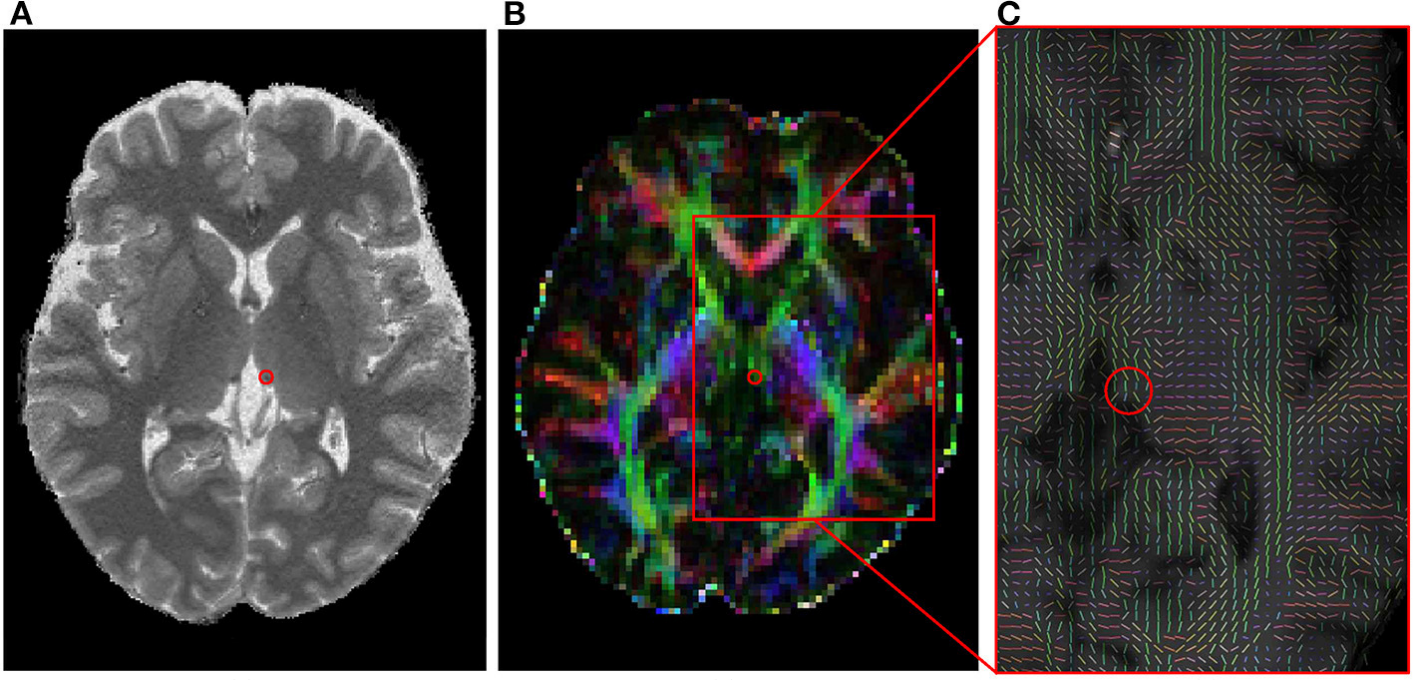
The habenula in a 3T MRI image and its diffusion tensor image. (A) A selected T1 image indicating a habenula area with a red circle. (B) A fractional anisotropy image corresponding to (A). (C) A zoomed fiber orientation image estimated with BEDPOSTX. The mean principle diffusion direction distribution in the red box of (B) was shown in a vector form. MRI, magnetic resonance imaging.
